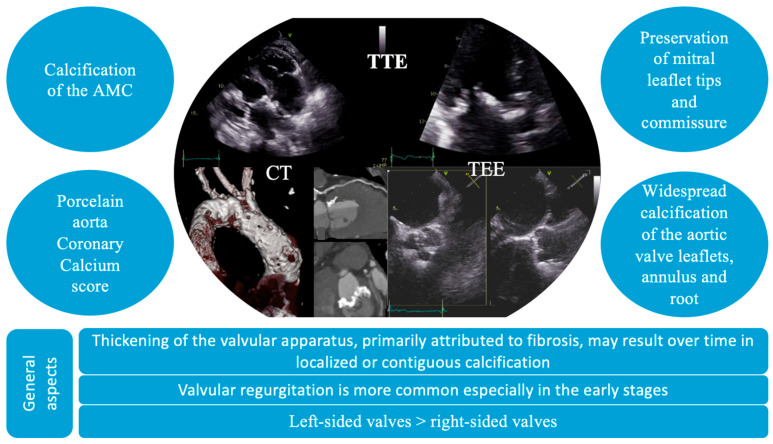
Particular imaging characteristics of radiation-induced valvular heart disease on multimodal imaging. AMC, aorto-mitral curtain; CT, computer tomography; TEE, transesophageal echocardiography; TTE, transthoracic echocardiography.
